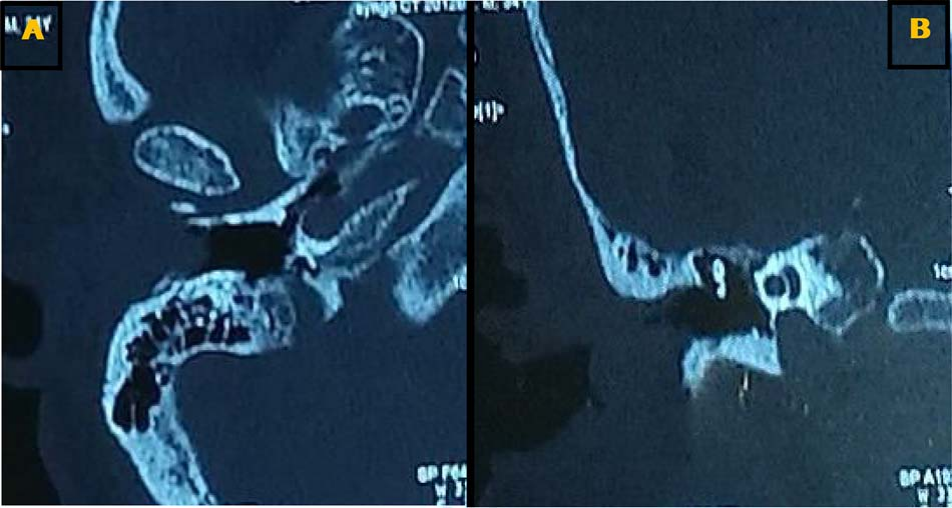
Axial (A) and coronal (B) CT scans showing a tissue lesion process of the external auditory canal with erosion of the eardrum and filling of a few mastoid cells.
